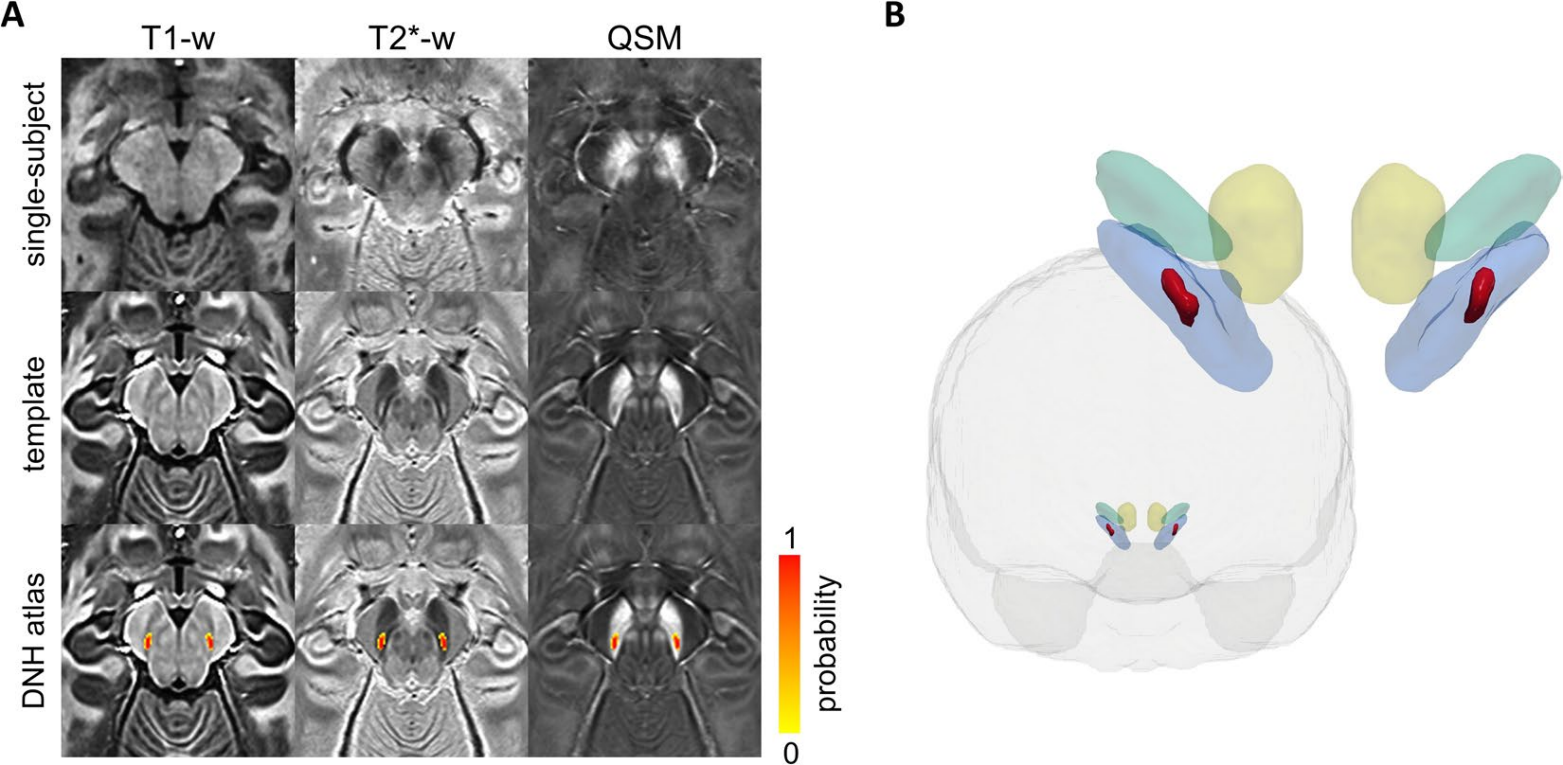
DNH atlas. (A) Single-subject images of a representative healthy volunteer (first row), study template (second row), and DNH probabilistic atlas overlaid onto the T1-weighted, T2*-weighted images, and the susceptibility map (third row) of the template. (B) 3D frontal view of the brain and the DNH ROI (red) obtained using a 0.5 probability threshold on the atlas. For anatomical reference, DNH is shown together with red nuclei (yellow), substantia nigra (blue), and subthalamic nuclei (green) from the PD25 atlas^18^ in the MNI152 2009b nonlinear asymmetric space.
